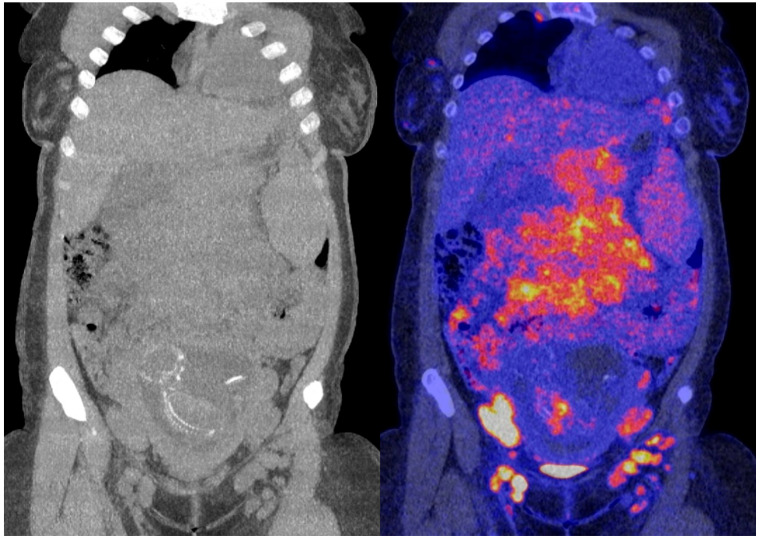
Coronal slice CT (left) and fused PET/CT images (right) of the fetus.
